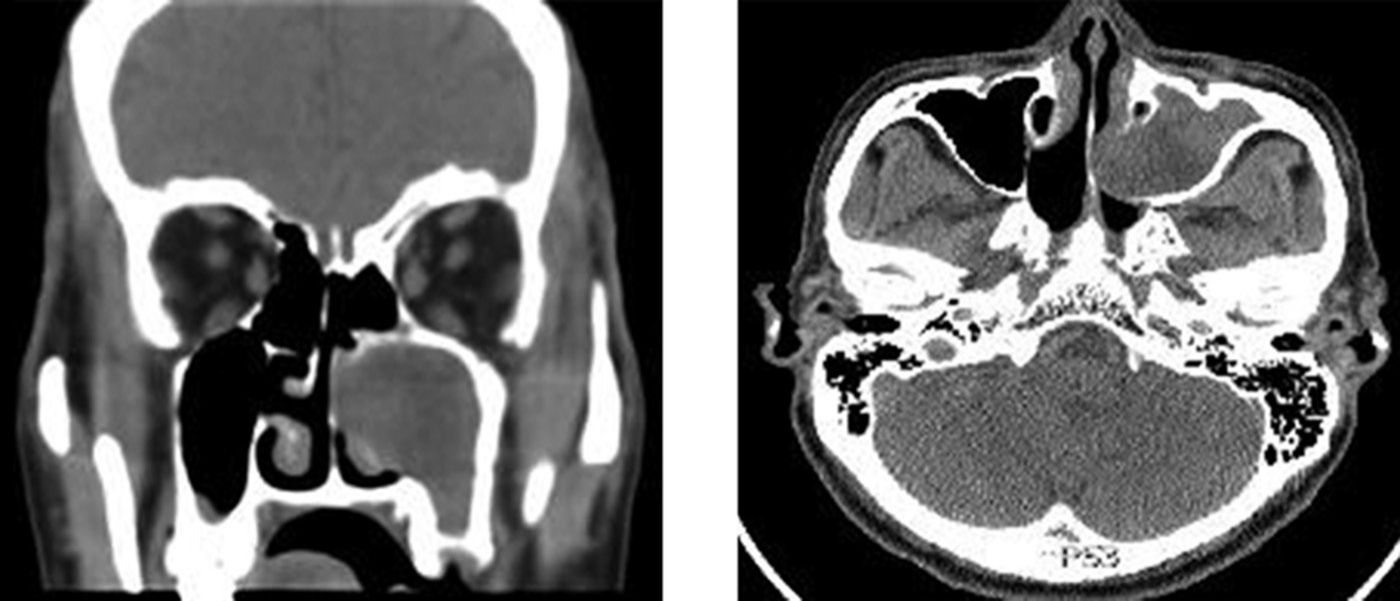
Computed Tomography Shows an Expansible Homogenous Opacity in the Left Maxillary Sinus Left: coronal plane; Right: axial plane.
